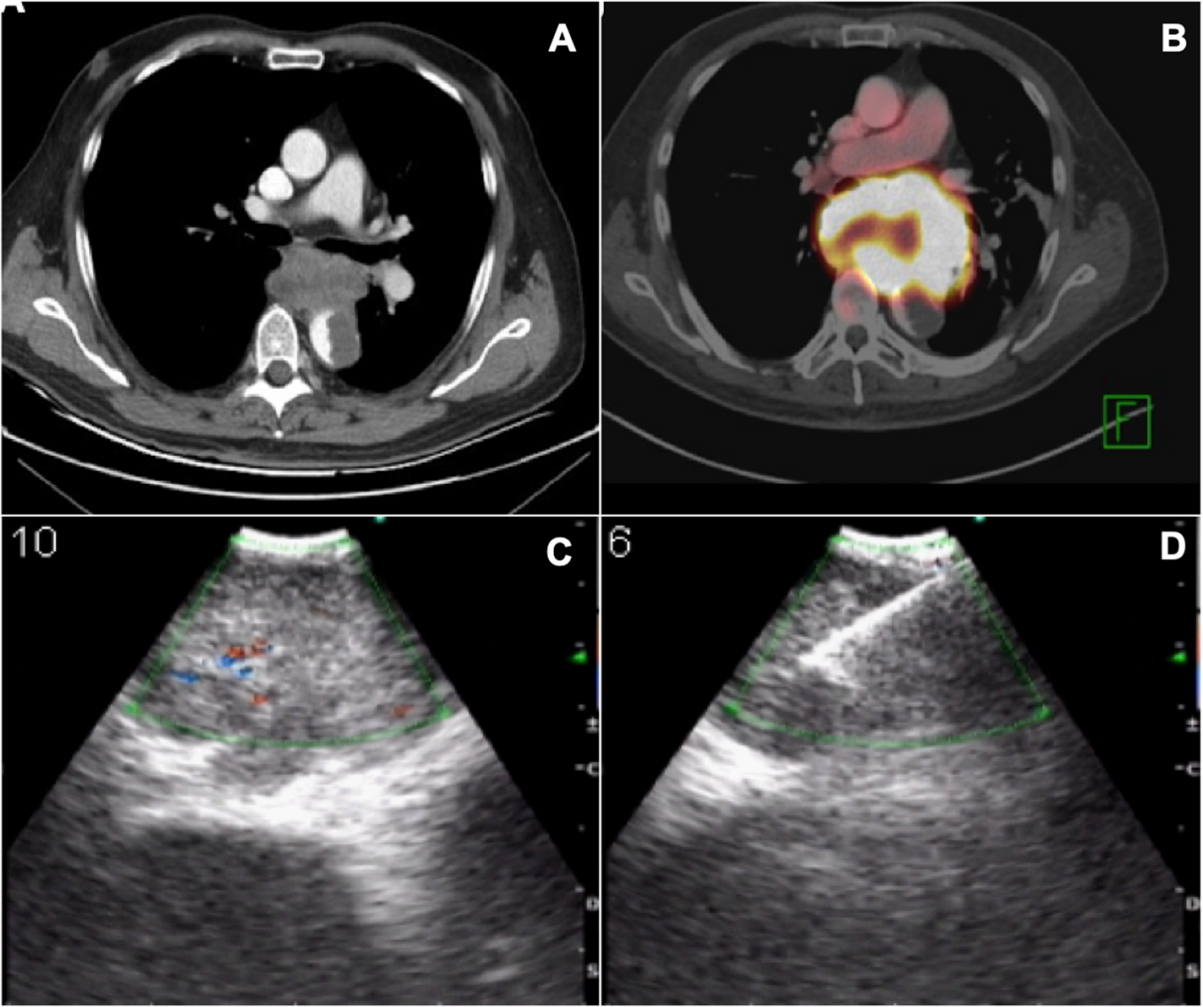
a Thorax computed tomography scan showing a mass in the posterior mediastinum that compresses the left main bronchus and infiltrates the aorta with obstruction greater than 50%. b Positron emission tomography-computed tomography scan showing hypermetabolic lesion that compresses the left main bronchus and infiltrates the aorta. c Endobronchial ultrasound showing a rounded lesion adjacent to the left main bronchus. d Image of the real-time fine needle puncture of the tumor for sampling
